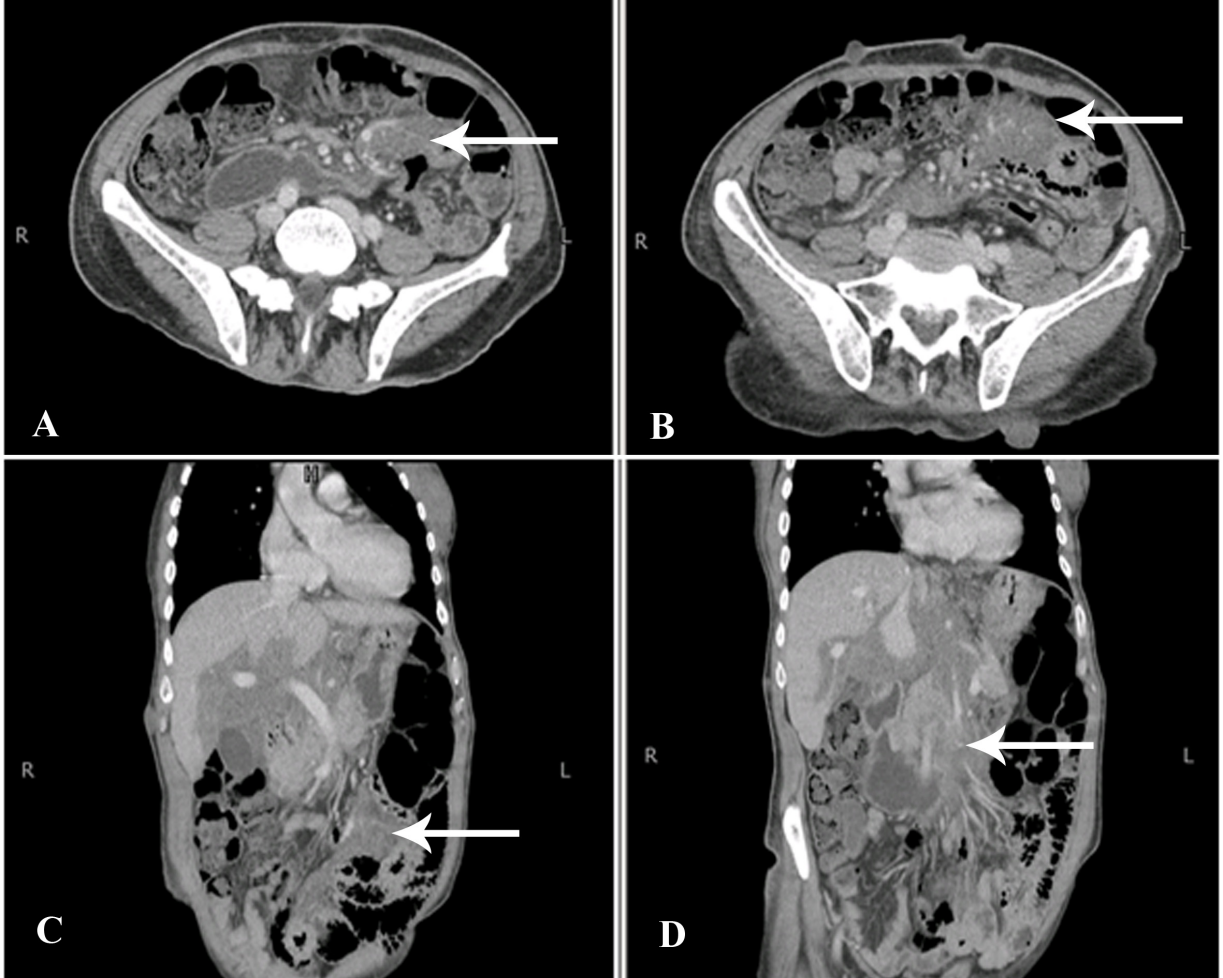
CT Abdomen Axial and Coronal Sections Extensive abdominal involvement of the mass was noted with extension along the superior mesenteric vessels into the mesentery (arrows). CT - computed tomography.
